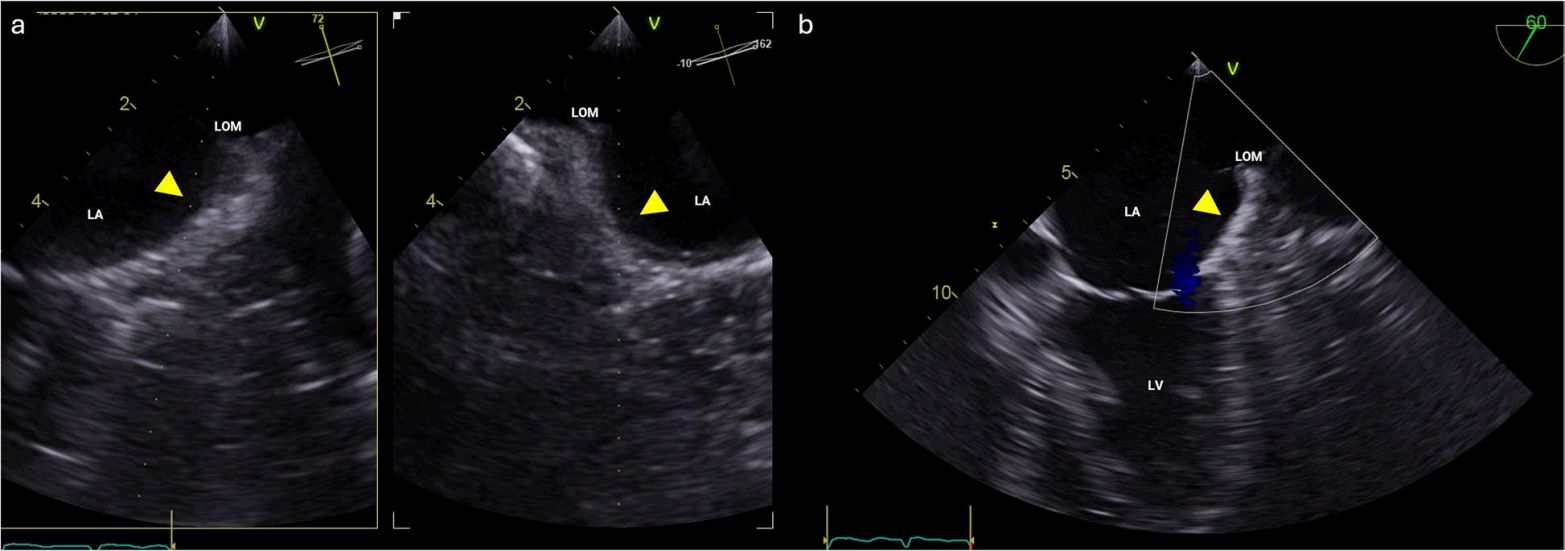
Initial three-dimensional (3D) transesophageal echocardiogram (TOE). A Magnified multiplane 3D TOE did not detect a patent left atrial appendage in its normal position (arrowheads). B Color Doppler unable to detect flow from the usual left atrial appendage location (arrowhead). LA, left atrium; LOM, ligament of Marshall; LV, left ventricle
